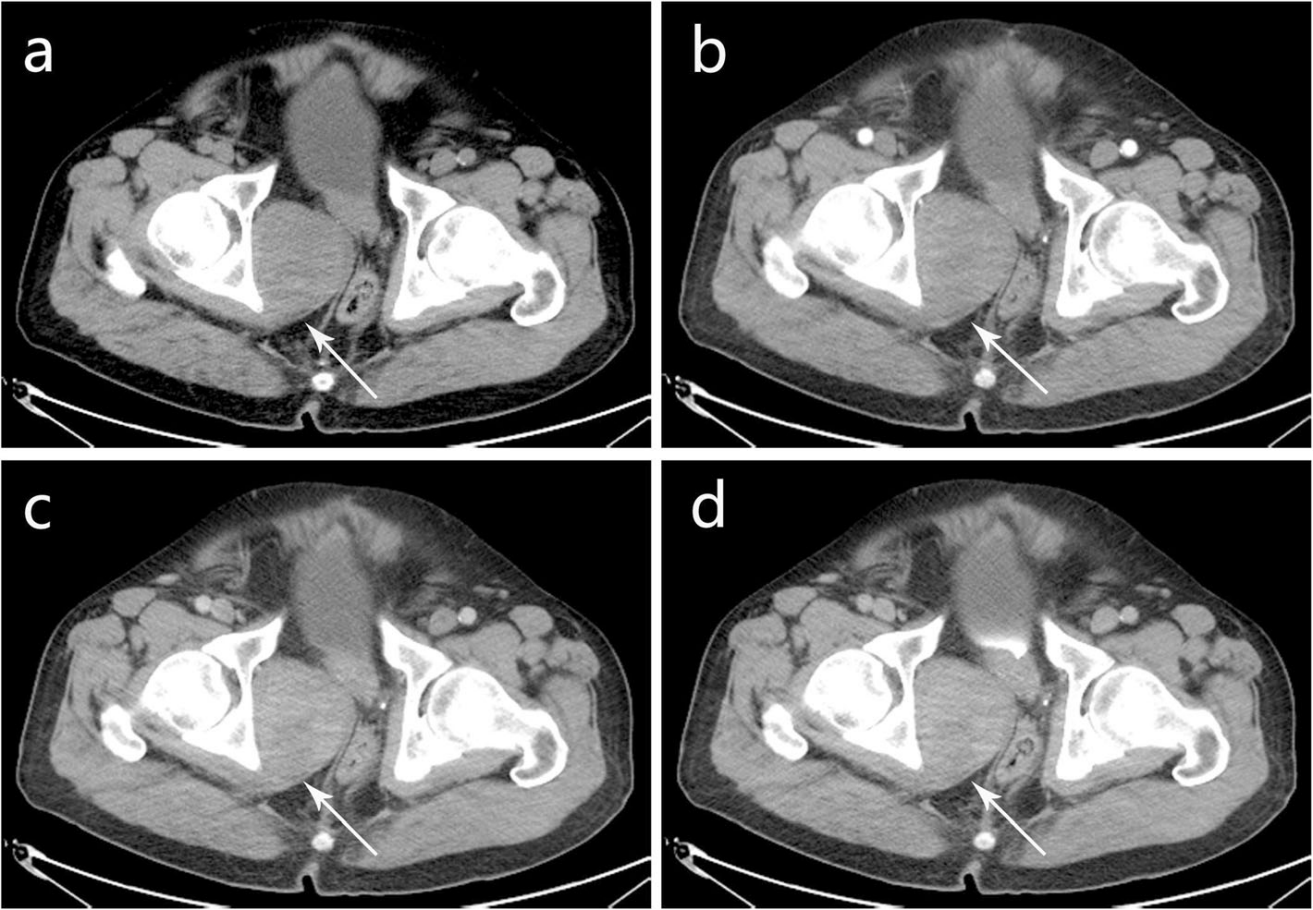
(a) Abdominal plain CT scan revealed an slightly heterogeneous soft tissue mass (white arrow) with well-defined boundary. The mass showed mild heterogeneous enhancement in (b) arterial, (c) venous, (d) and delayed phase
